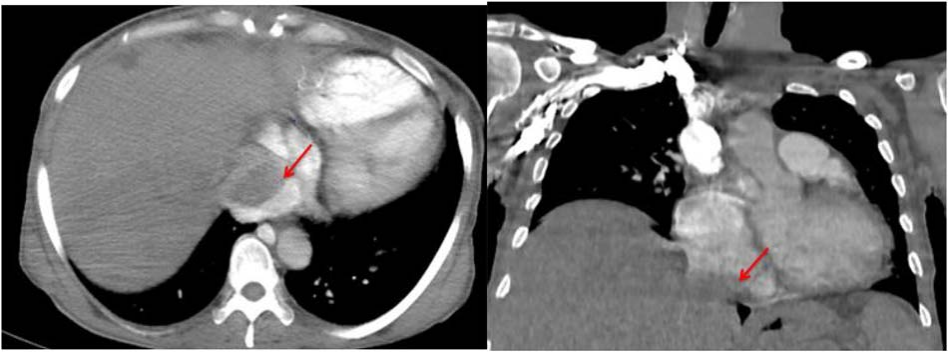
Angio-CT thoracic images reveal the presence of a mass in the inferior vena cava extending into the right atrium (arrow).
